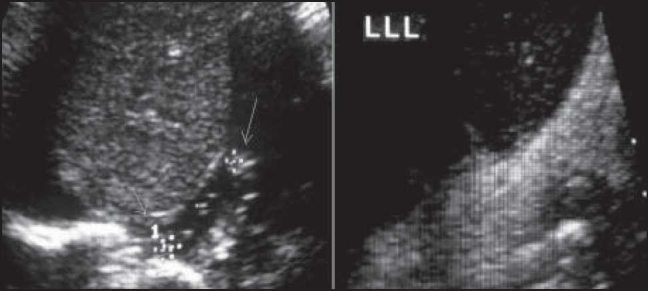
Measurement of the intra-abdominal portion of esophagus using left liver lobe as an ultrasonic window
